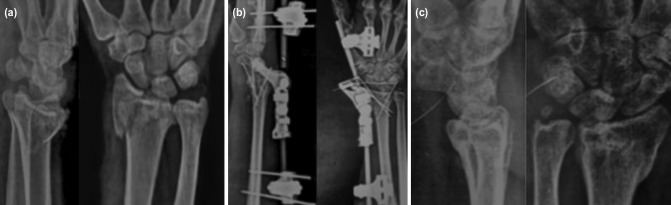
Radiographs of right wrist (a) pre-operative, (b) post-operative, (c) after 12 months.
